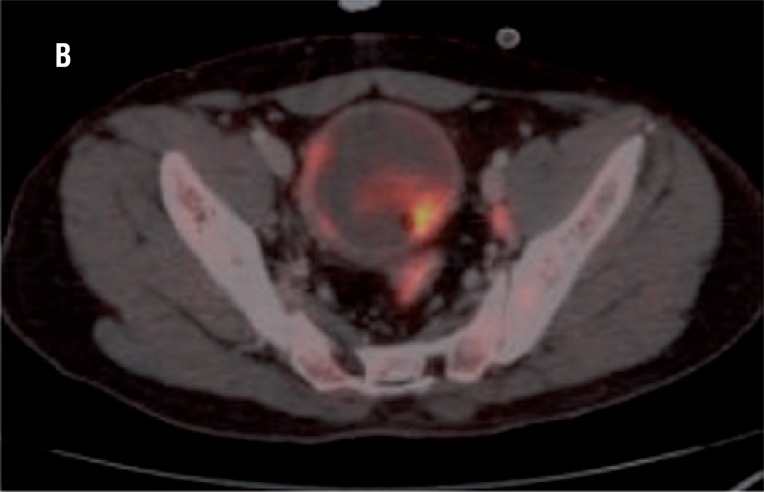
PET / CT showing moderate FDG uptake in left internal iliac nodes (arrow).
